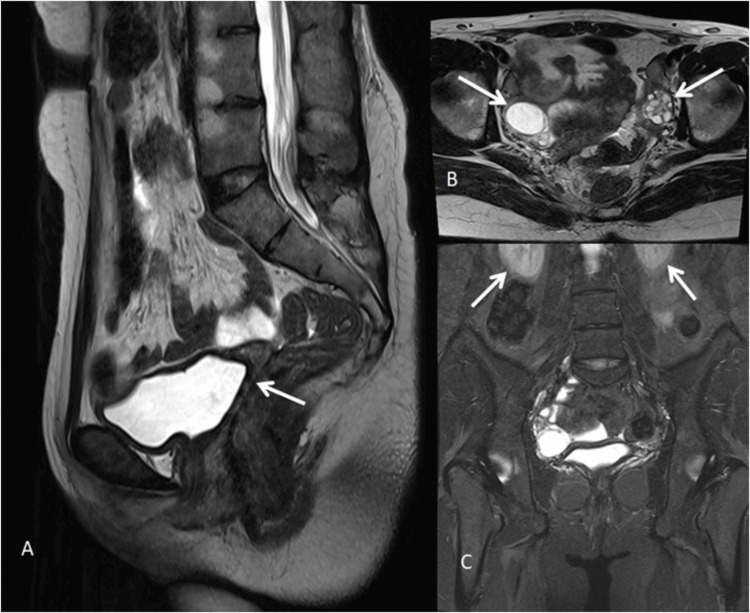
Mayer-Rokitansky-Küster-Hauser syndrome type A (A) Sagittal T2-weighted image demonstrating the absence of the uterus, cervix, and upper two-thirds of the vagina (white arrow), consistent with Müllerian agenesis. (B) Axial T2-weighted image showing normal bilateral ovaries with follicles (white arrows). (C) Coronal STIR image demonstrating normally located kidneys in the renal fossae (white arrows). STIR: Short tau inversion recovery.
